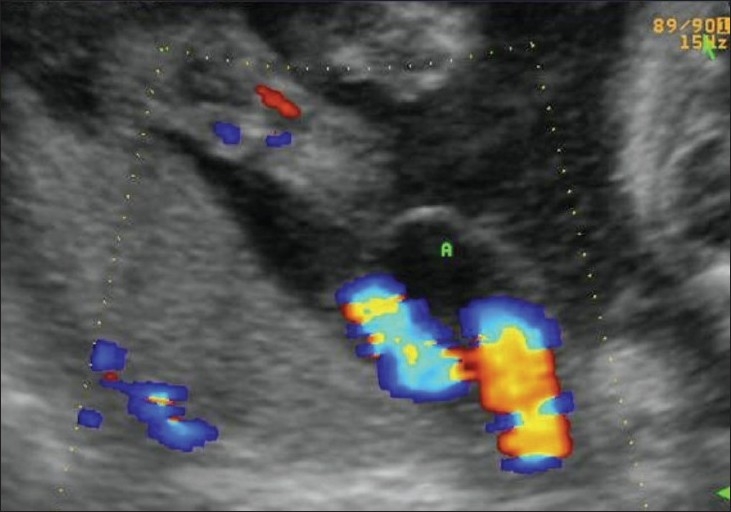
Allantoic cyst. Color Doppler helps differentiate between an allantoic cyst (A) and an umbilical cord aneurysm
